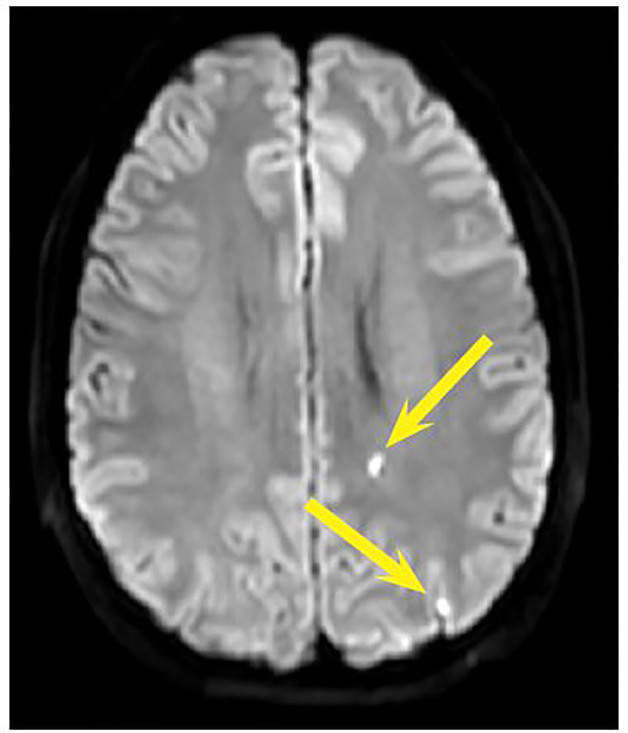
MRI transverse view showing areas with small ischemic lesions (yellow arrows).
